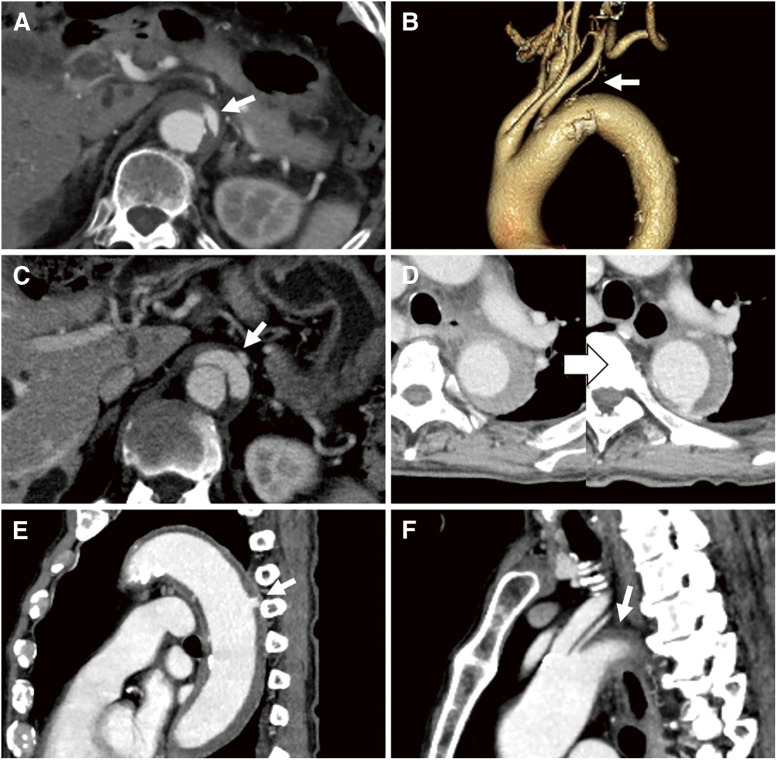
Fig. 1 Preoperative computed tomography. (A) Computed tomography on the day of admission reveals a tear in the aortic dissection at the origin of the celiac artery. (B) Computed tomography shows the shared origin of brachiocephalic and left common carotid arteries. The left vertebral artery arising directly from the aortic arch on the distal side of the left subclavian artery is indicated with an arrow. (C) Computed tomography performed on the 11th day post-admission indicates dilation of the false lumen. (D) Computed tomography performed on the day of admission and the 11th day post-admission (left to right) indicates enlargement of the descending aorta from 43 to 48 mm. (E) Computed tomography performed on the 11th day post-admission reveals an ulcer-like projection in the descending aorta. (F) Computed tomography performed on the 11th day post-admission reveals that the intramural hematoma with ulcer-like projection extended close to the left subclavian artery and isolated left vertebral artery.
